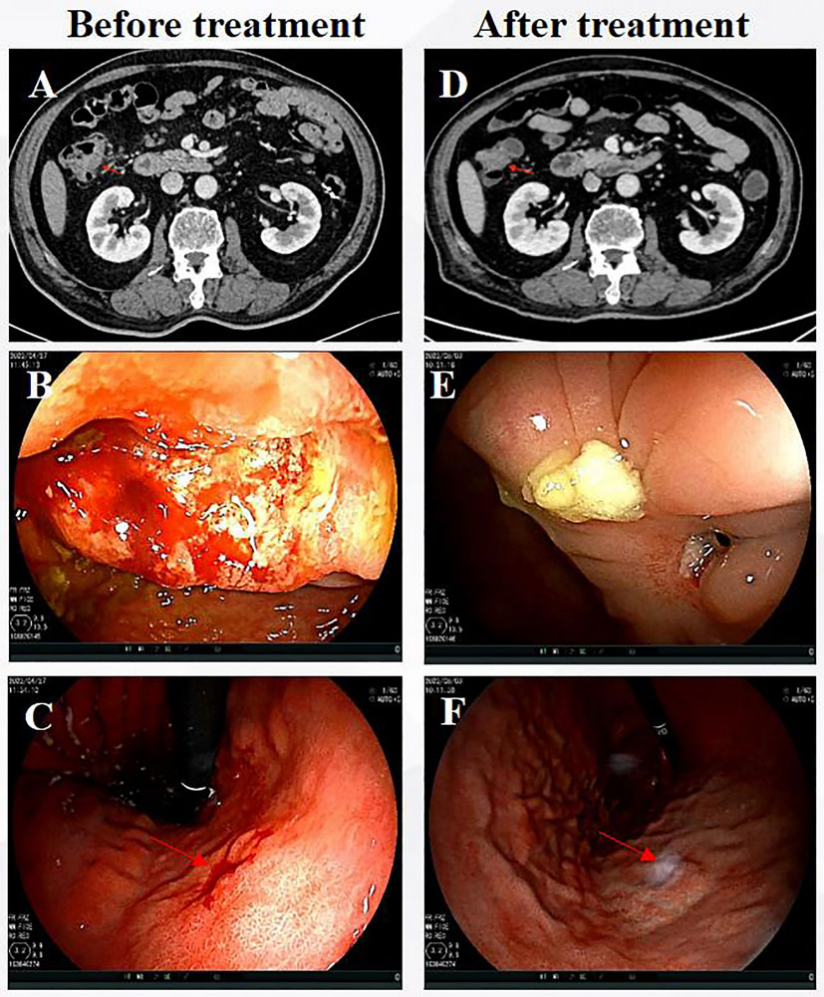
Imaging and endoscopic results before and after neoadjuvant treatment with trastuzumab, pertuzumab, and chemotherapy. (A) CT image of colon lesion before treatment, (B) colonoscopic image of colon cancer before treatment, (C) gastroscopic image of gastric cancer before treatment, (D) CT image of colon lesion after treatment, (E) colonoscopic image of colon cancer after treatment, and (F) gastroscopic image of gastric cancer after treatment.
